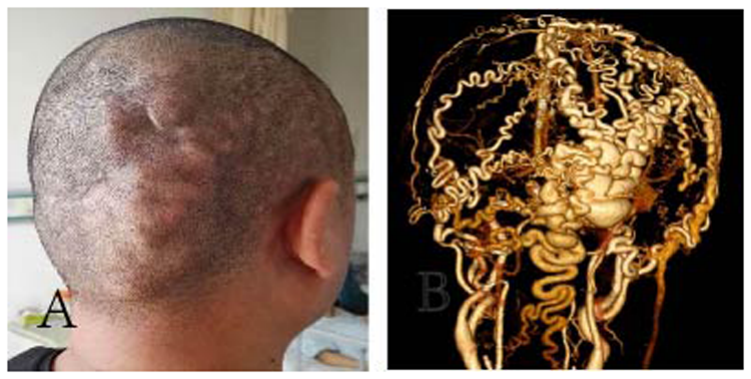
Scalp arteriovenous fistula (S-AVF). (A) 41-year-old man presented with a pulsating mass measuring 12 × 7 × 3 cm in size in his right occipital part. (B) CTA shows a complex multiple arteriovenous fistula located in the right occipital scalp and part of the right temporoparietal region, blood was supplied by branches of bilateral external carotid arteries and drained by the right superficial occipital vein.
